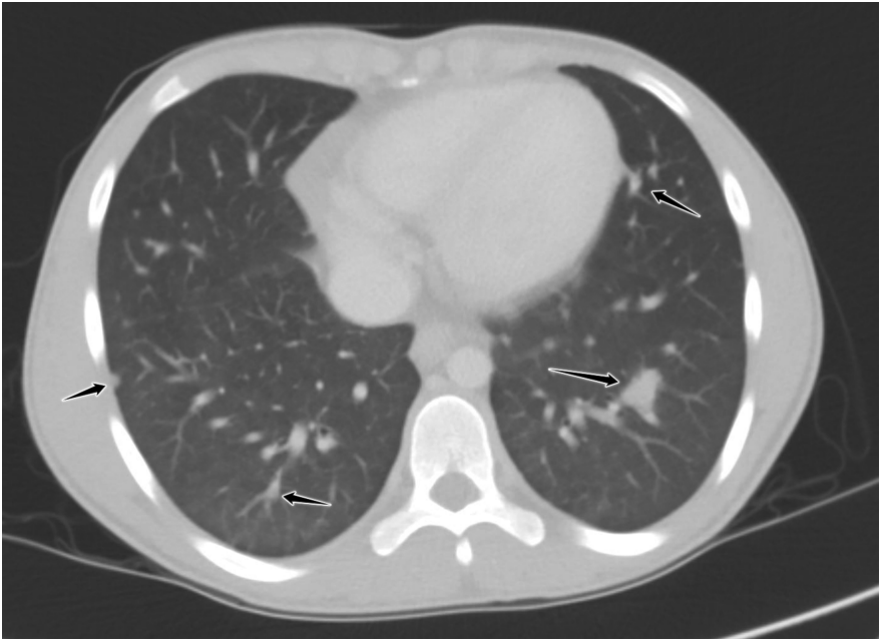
Axial reformatted, lung window CT image through the lower chest demonstrating multiple noncalcified, well-demarcated pulmonary nodules throughout all lobes (black arrows).
